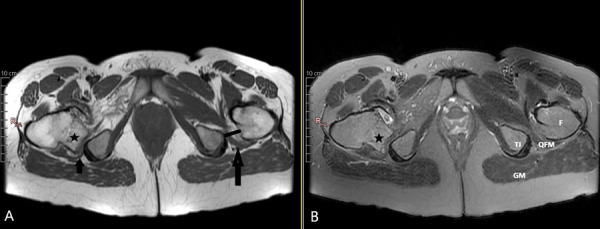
Hip MRI examination of the same patient. Fat saturation FSE T2W axial image (A) and FSE T1W axial image (B). *Osteochondroma* (asterisk) with a 6 mm cartilage cap that causes severe QFM compression by narrowing the right *ischiofemoral distance*. Normal *ischiofemoral distance* and QFM on the left. *GM: Gluteus maximus, TI: Tuber ischium, QFM: Quadratus femoris muscle, F: Femur.*
